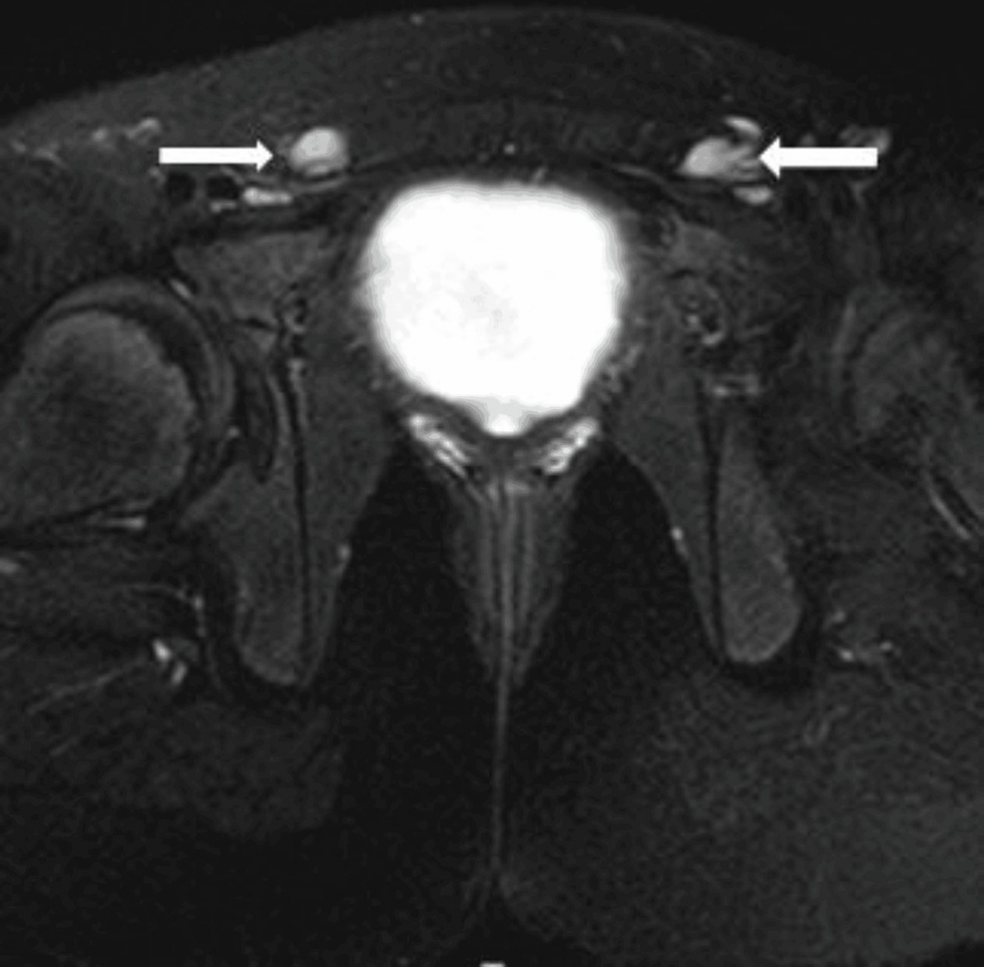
T2-weighted MRI of the pelvis. The axial view shows bilateral testicular tissue in the inguinal canals in the index case (the white arrows).
